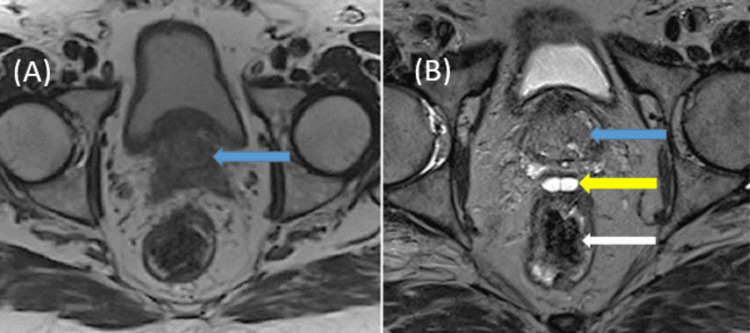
Repeat axial (A) and (B) T2 MRI sequences showing visualisation of the prostate (blue arrow), hydrogel barrier (yellow arrow) and rectum (white arrow) following cessation of curcumin supplements one week later.
